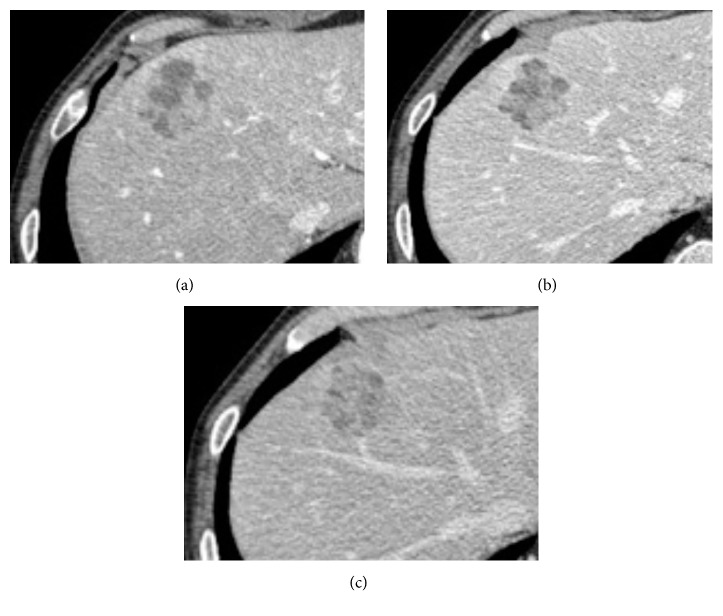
Images of contrast abdominal computed tomography (CT). CT showed that the tumor was slowly enhanced from the early phase to the parallel phase. (a) Early phase. (b) Delayed phase. (c) Parallel phase.
